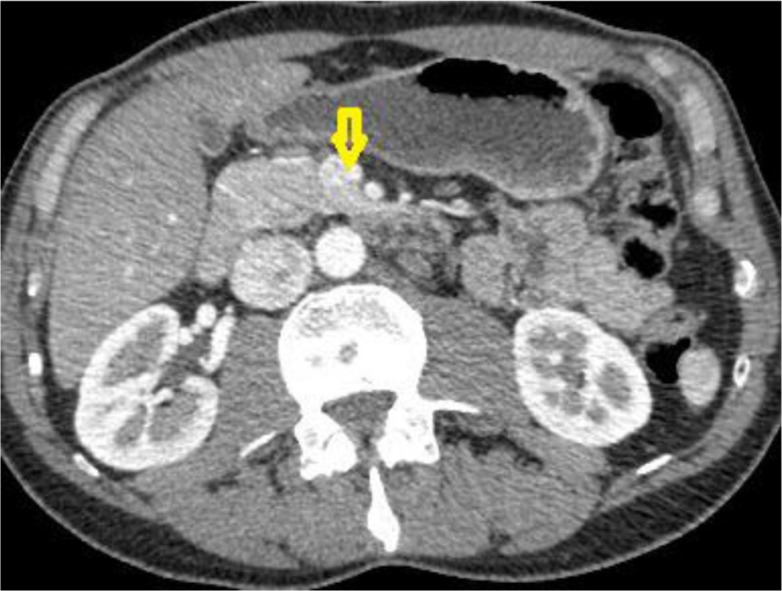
CT Abdomen showing lesions in the pancreas.
